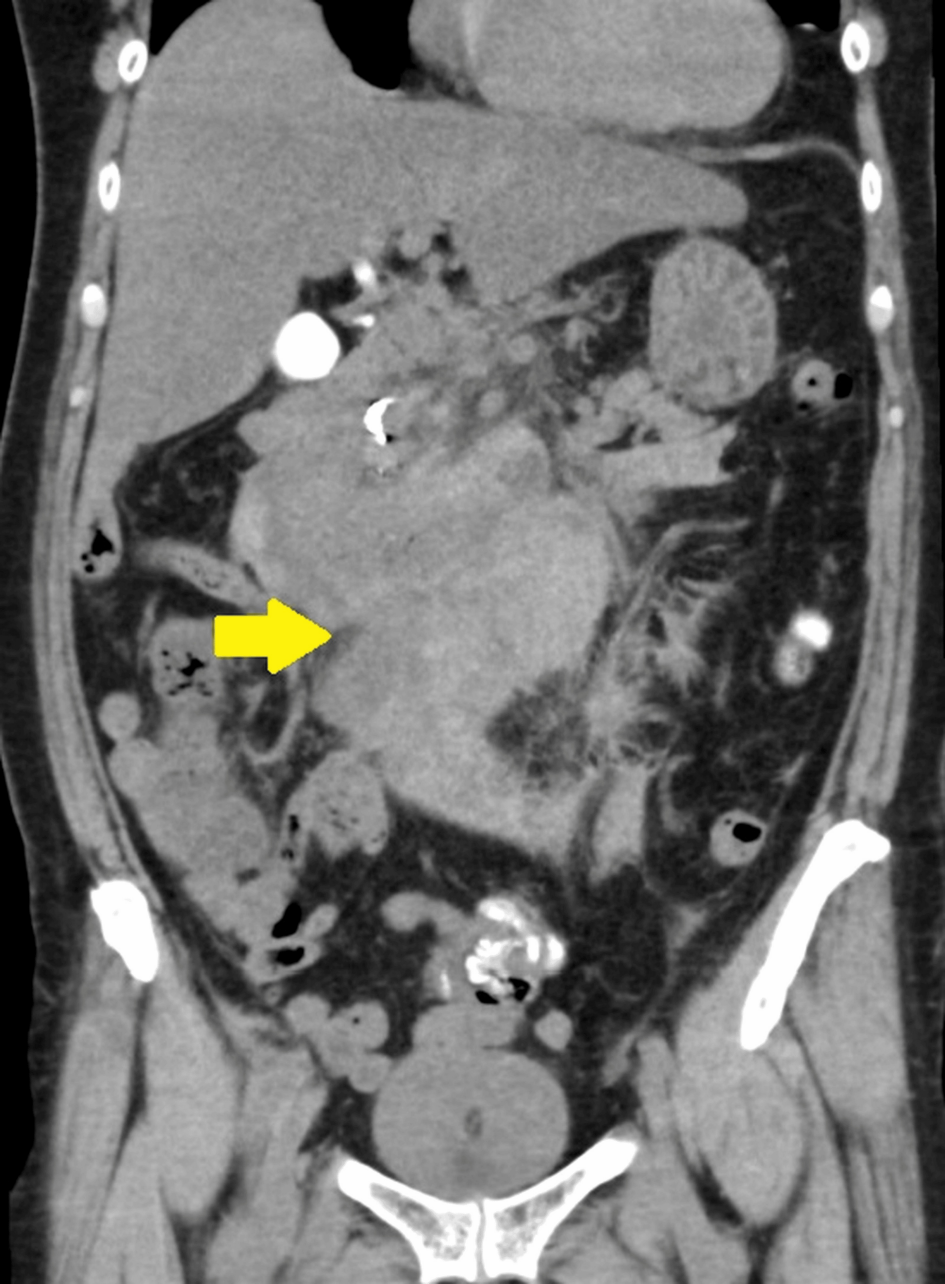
Ruptured pancreaticoduodenal artery aneurysm due to arch-ligament compression syndrome Subsequent computed tomography (CT) imaging revealed inflammation extending from the duodenum to the pancreas with hemoperitoneum, raising concerns for acute pancreatitis or pseudoaneurysm rupture (the yellow arrow).
